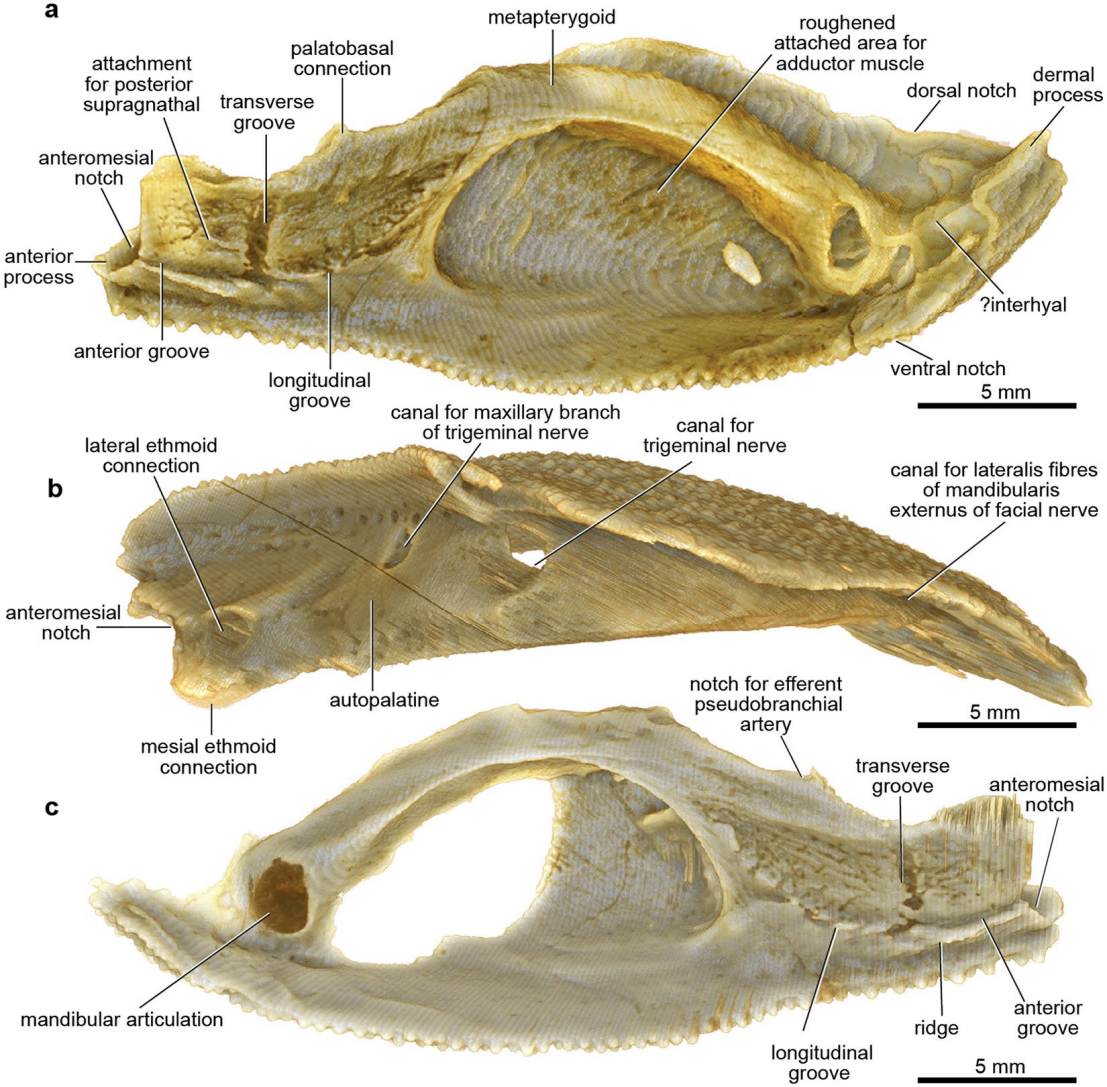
Dermal cheek unit with attached perichondrally ossified cartilage elements of articulated buchanosteid arthrodire (ANU V244) based on high-resolution CT. (a,b) Right suborbital and postsuborbital plates, with attached palatoquadrate and? interhyal, in internal (a) and dorsal (b) views. (c) Left suborbital plate (incomplete) and attached palatoquadrate in internal view.
